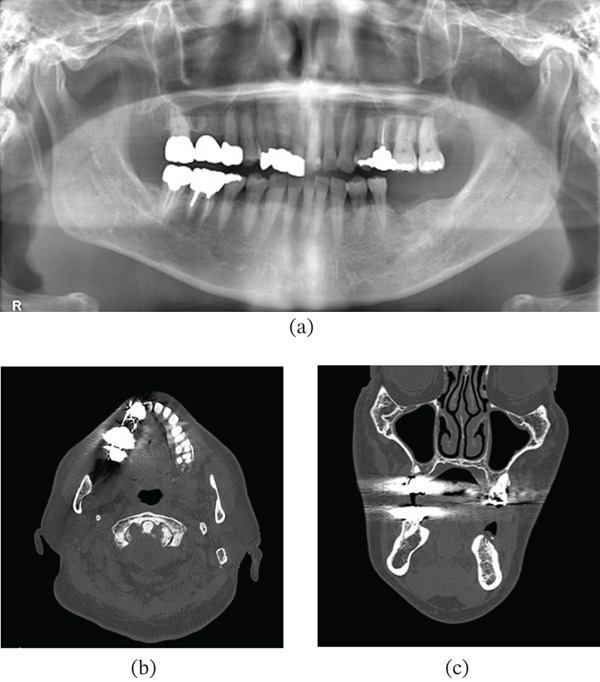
Imaging findings at initial presentation. (a) Orthopantomogram shows no lesion‐specific findings in the left maxillary second premolar region. Noncontrast computed tomography images ((b) axial; (c) coronal) show no resorption of the adjacent maxillary bone.
